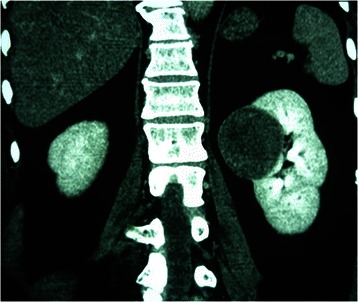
Contrast-enhanced CT image revealed heterogeneous and varying degrees of enhancement.
